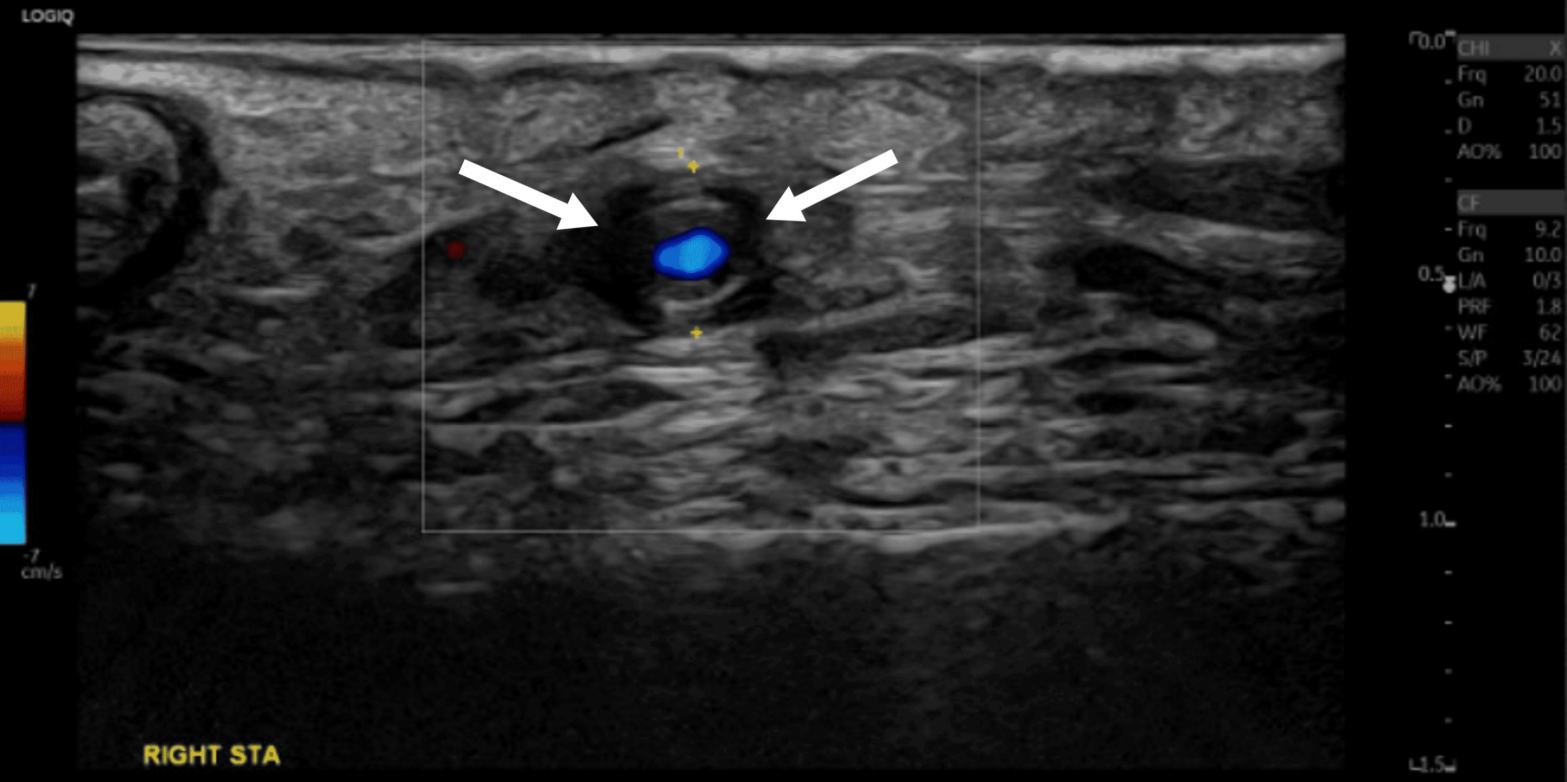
Temporal artery color Doppler ultrasound (right STA) Ultrasound images show a dark hypoechoic halo around the right STA lumen (arrows), representing the vessel wall inflammation STA: superficial temporal artery
